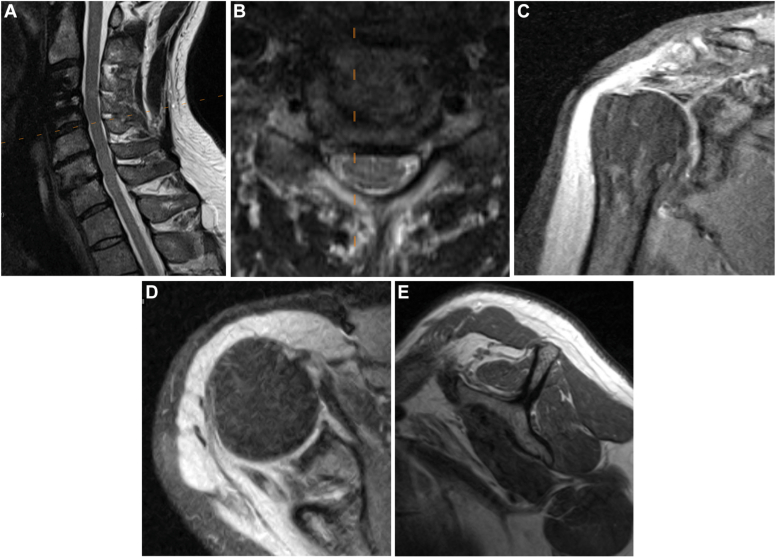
(A-E) MRI from clinical vignette #3. *MRI*, magnetic resonance imaging.
